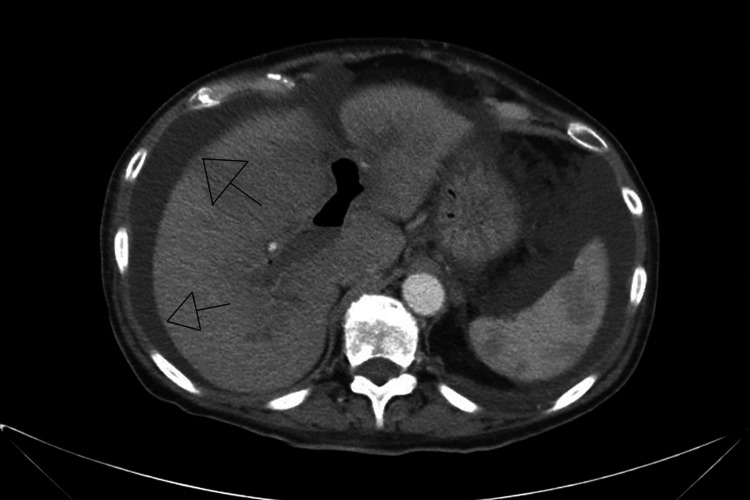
Liver abscess seen in an abdominal CT scan
